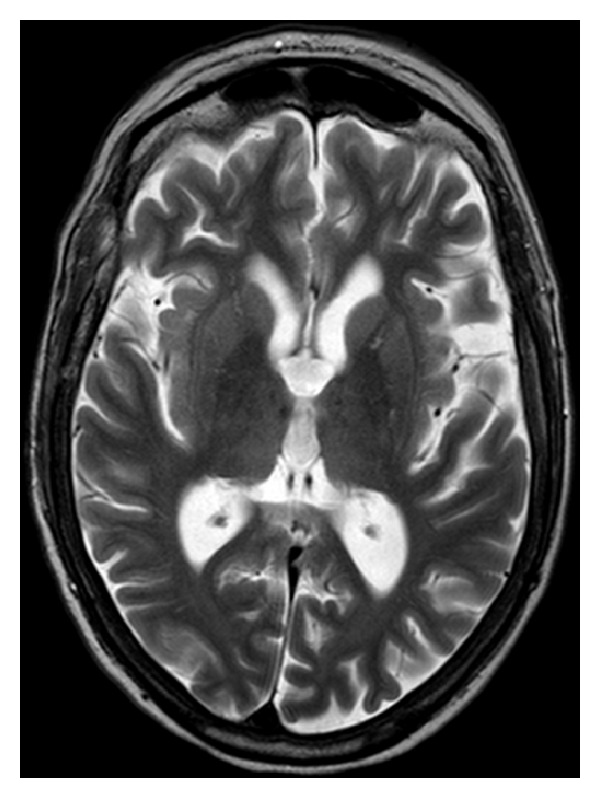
MRI brain showing CSF spaces and cerebral atrophy that are prominent for a patient of this age.
